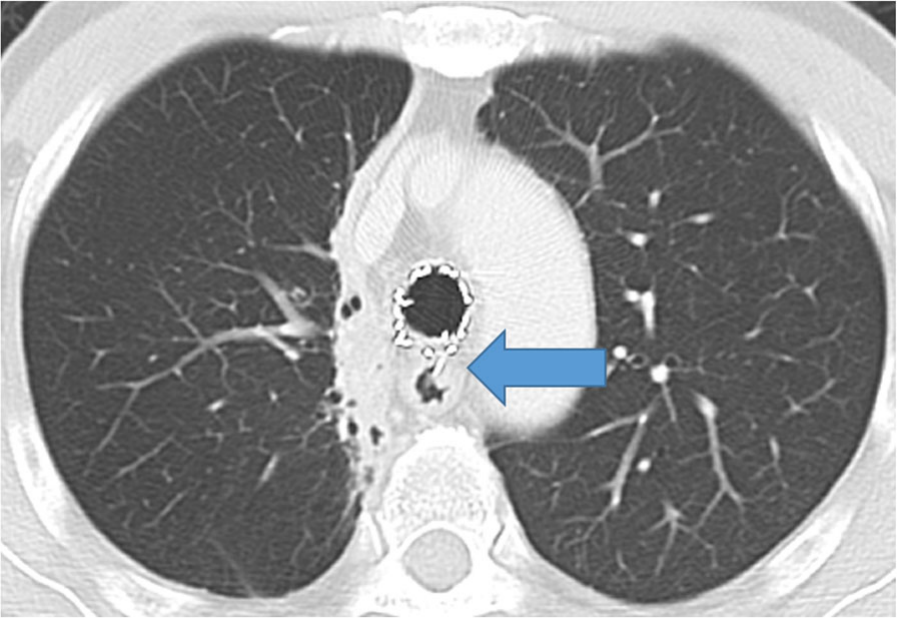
Preoperative chest computed tomography (CT) scan shows that a part of the broken tracheal stent has penetrated into the esophagus
